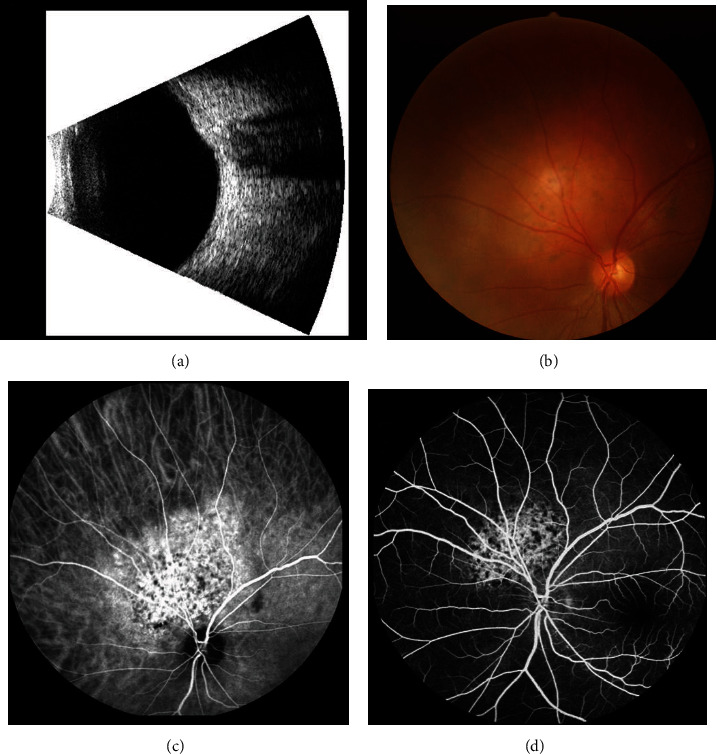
An example of RCC patients with IOM. Notes: (a) ophthalmic B-type ultrasound; (b) fundus photography; (c) indocyanine green angiography; and (d) fundus fluorescein angiography. Abbreviations: RCC: renal cell carcinoma; IOM: intraocular metastasis.
